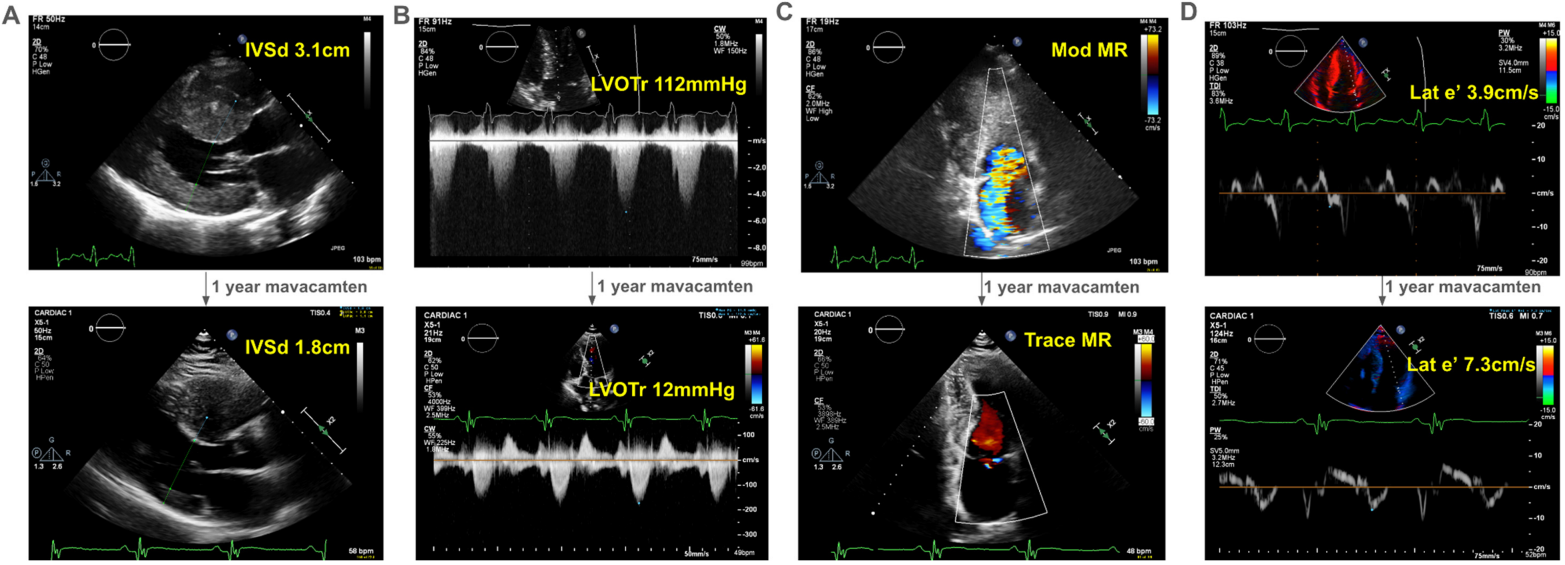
Echocardiographic changes after one-year of mavacamten in a 41-year old man with obstructive hypertrophic cardiomyopathy. With one-year of treatment with mavacamten, our proband experienced improved symptoms, which correlated echocardiographically (see Supplementary Video S1) to (A) a decrease in interventricular septum thickness on parasternal long axis view, (B) his left ventricular outflow tract gradient at rest, (C) the degree of mitral regurgitation, and (D) his diastolic function as represented by his lateral e’. IVSd, interventricular septum thickness in diastole in cm; LVOTr, left ventricular outflow tract gradient at rest in mmHg; MR, mitral regurgitation; lat e’, lateral e’ in cm/s, a spectral Doppler measurement reflecting diastolic function.
